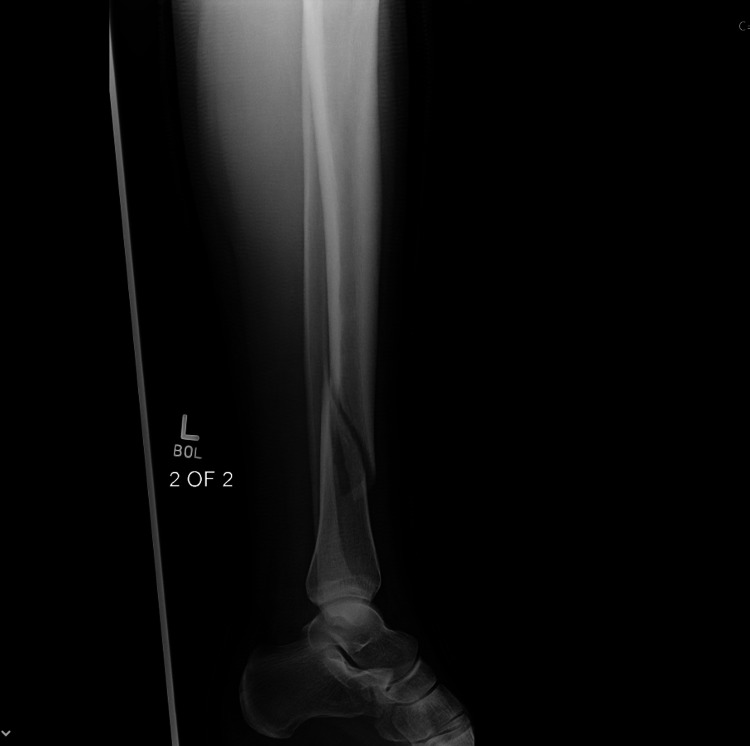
Distal Tibia fracture at the time of initiation of LIPUS therapy (6 months post injury - lateral view) LIPUS - Low-Intensity Pulsed Ultrasound
